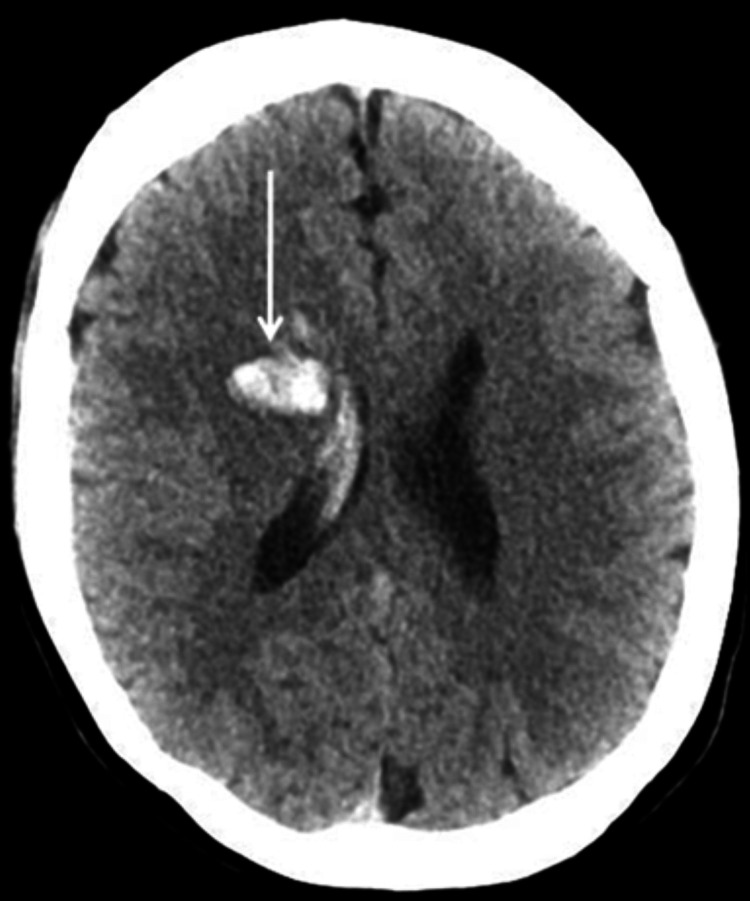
CT showing a right frontal capsular parenchymal hemorrhage (arrow), with rupture into the right lateral and third ventricles CT, computed tomography
